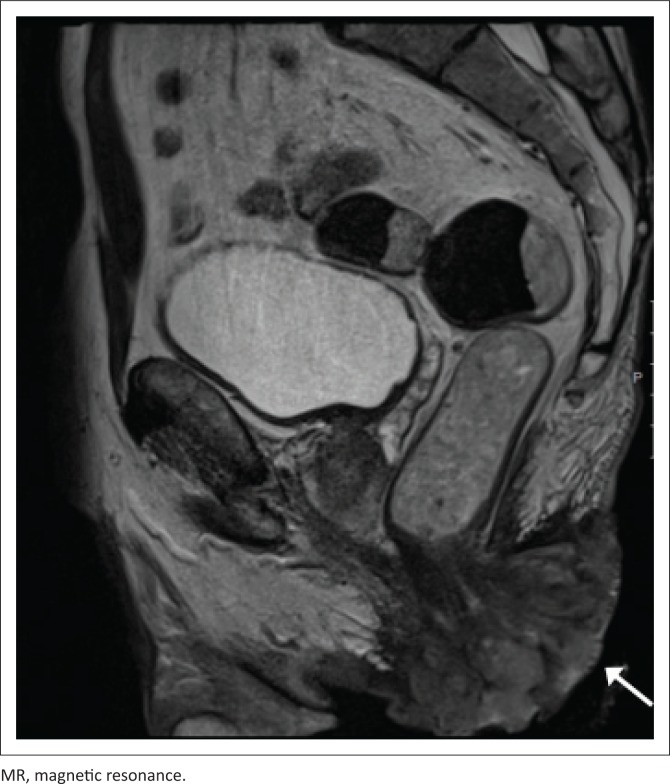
Sagittal T2-weighted MR imaging of a 42-year-old male patient (as shown in Figure 5), demonstrating a large anal soft tissue mass (arrowed) of intermediate signal intensity, with local soft tissue invasion.
